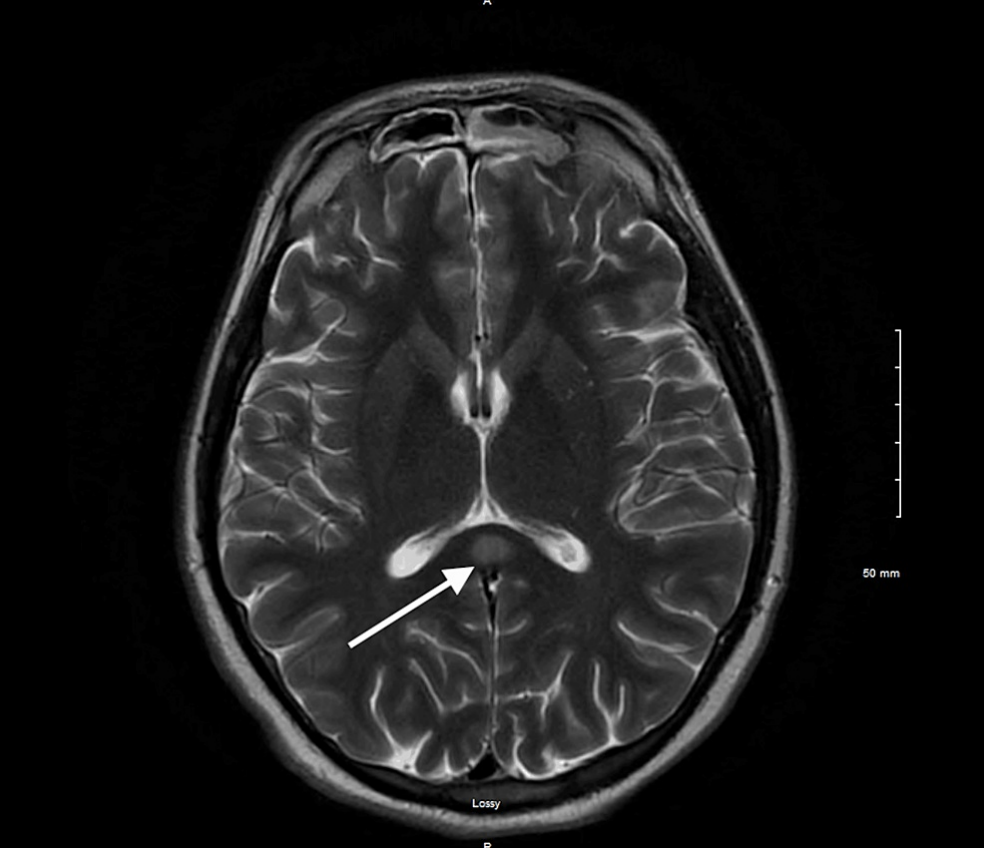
T2-weighted axial MR image showing symmetrical hyperintensity in the region of the corpus callosum, particularly concentrated in the splenium
